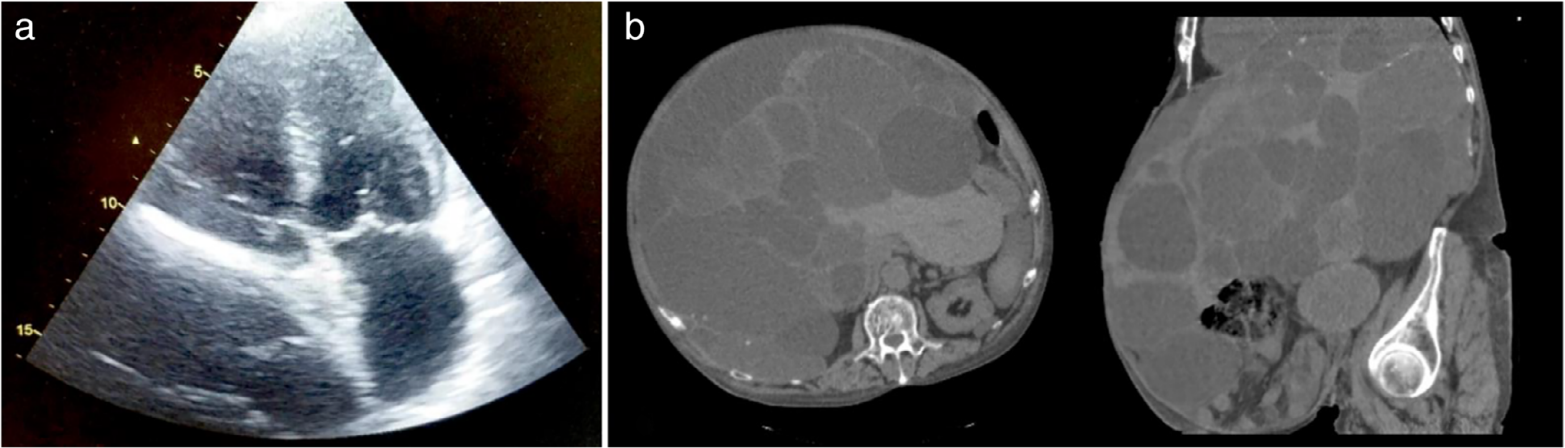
(a) Bed‐side cardiac ultrasound (apical 4‐chamber) showed a right atrial compression, caused by massive liver cysts; (b) computed tomography showed the presence of multiple liver cysts and their mass effect.
